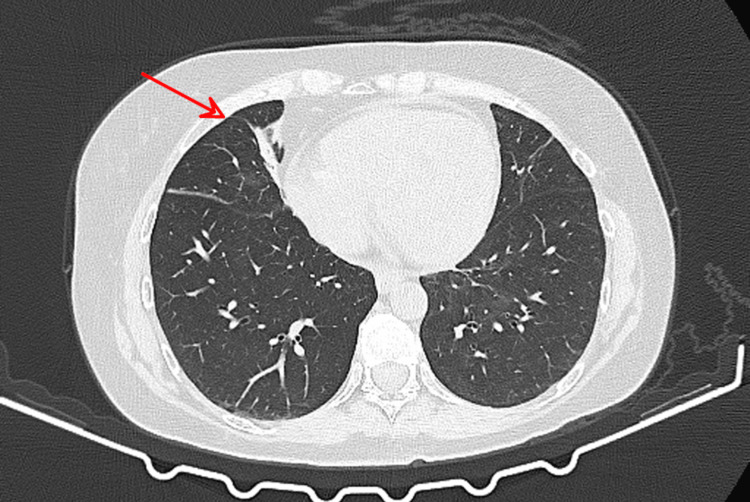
Follow-up chest CT 43 days after pembrolizumab administration Marked resolution of previously noted pulmonary abnormalities, with residual band-like subsegmental atelectasis in the right middle lobe (red arrow), representing a pre-existing finding. CT: computed tomography
